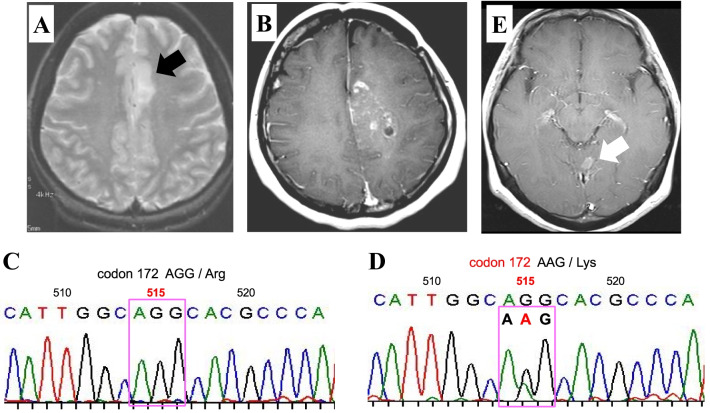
Representative patient treated with reirradiation and temozolomide (ReRT/TMZ) showing the usefulness of *IDH1/2* mutational status evaluation in establishing the RIG diagnosis (Case 9). A T2-weighted magnetic resonance image at the initial presentation showing a hyperintense lesion in the left medial frontal lobe (black arrow). B T1-weighted magnetic resonance image with gadolinium enhancement obtained 17 years after the primary tumor showing a contrast-enhanced lesion just posterior to the primary tumor, which was included within the prior radiation field. C Sanger sequencing analysis of the secondary tumor (glioblastoma) showing the homozygous G nucleotide at codon 515 of the *IDH2* gene, indicating the *IDH2* gene was wild-type. D Sanger sequencing analysis of the primary tumor (diffuse astrocytoma) showing the heterozygous G and A nucleotides at codon 515 of the *IDH2* alleles, indicating the *IDH2* gene was mutant. E T1-weighted magnetic resonance images with gadolinium enhancement were obtained 23.0 months after the secondary tumor diagnosis showing a distant recurrence in the cerebellum (white arrow)
